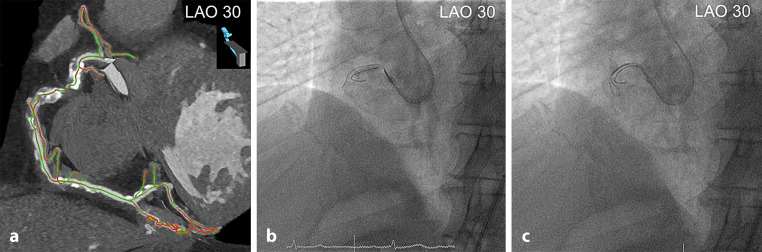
a–c Coronary computed tomography angiography (*CTA*) for guiding balloon-assisted subintimal entry. Colour-coded CTA multiplanar reconstruction showing the exact course of severely calcified and tortuous chronic total occlusion of the right coronary artery (*RCA*) with adequate length of the proximal segment of the RCA for balloon-assisted subintimal entry. The coronary segments are displayed in *green* (no foreshortening) or *red* (substantial foreshortening) (a). Based on CTA reconstructions aligned with the angulation of the C‑arm, a 3.0 × 15 mm balloon was inflated in the proximal RCA, and a Fielder XT‑A guidewire (Asahi Intecc, Nagoya, Japan) was advanced into the subintimal space (b). After balloon deflation, the Turnpike Spiral microcatheter (Teleflex, Wayne, PA, USA) was advanced, and a knuckled Fielder XT‑A guidewire was pushed into mid-RCA (c). *LAO* left anterior oblique
